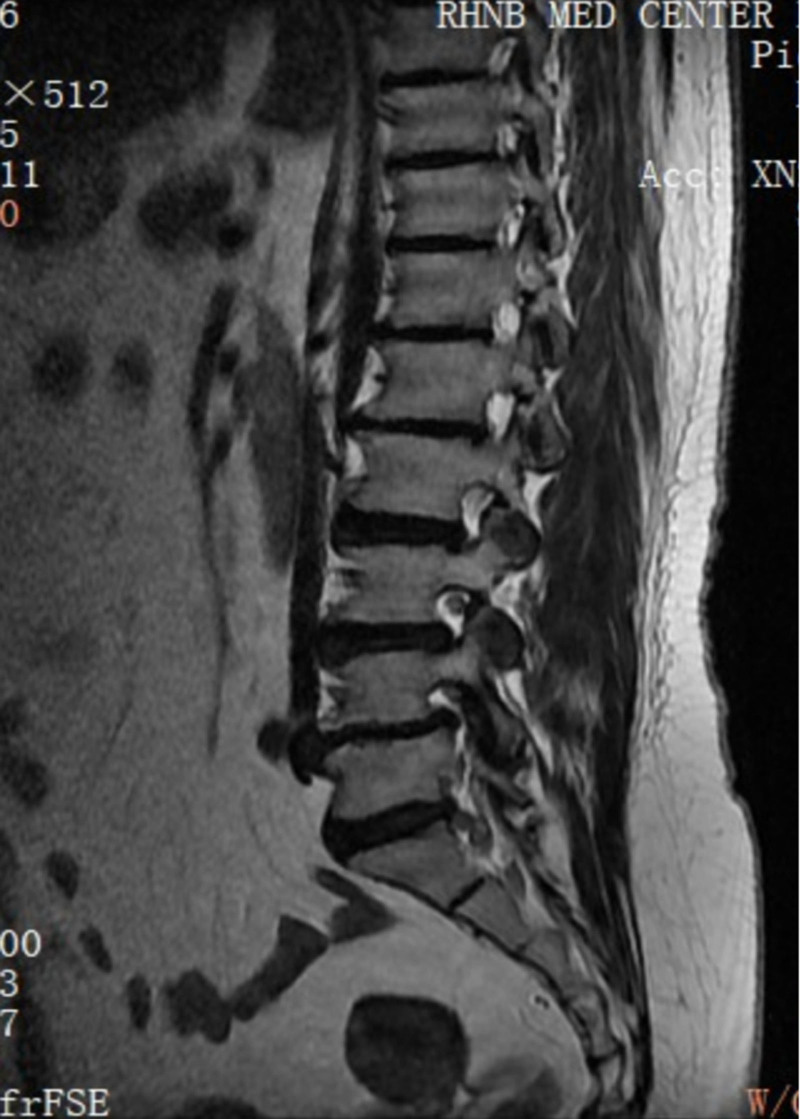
MRI sagittal view showing migrated lumbar disc herniation at the L5/S1 level. MRI = magnetic resonance imaging.
